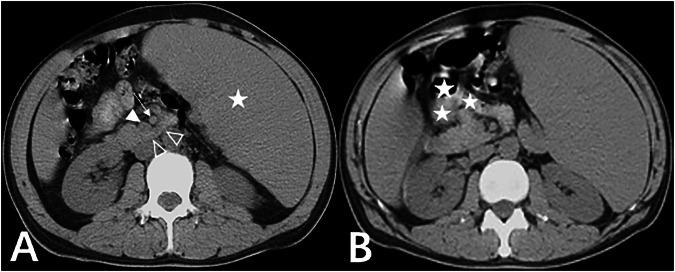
Fourty-five-year-old man with megalosplenia and jejunal transposition. A Axial CT scan showed the normal path of the duodenum horizontal segment (hollow triangle) behind the SMA (thin arrow), the normal relationship of the SMA/SMV (solid triangle), the space normally occupied by the jejunum instead occupied by the enlarged spleen (star). B Axial CT scan showed the jejunum (star) flipped across the front of mesenteric blood vessels to the right abdomen
